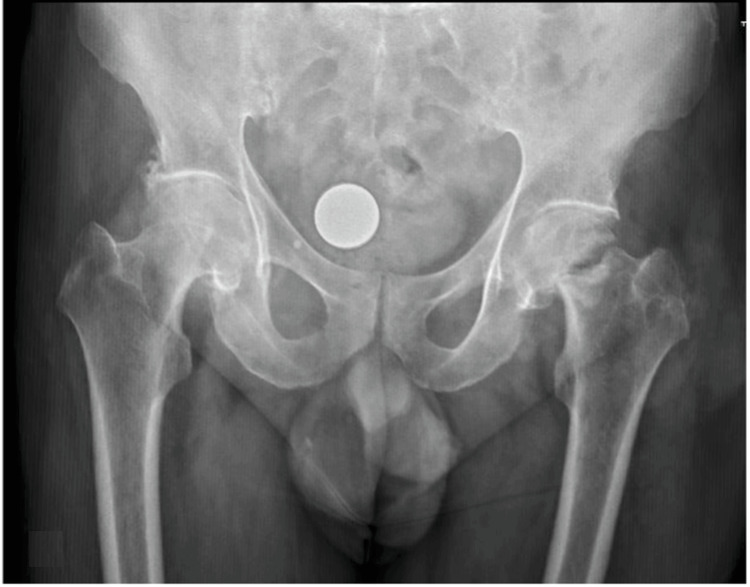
Anteroposterior pelvic radiograph, performed with traction and internal rotation of the limbs, showing an old left femoral neck fracture
